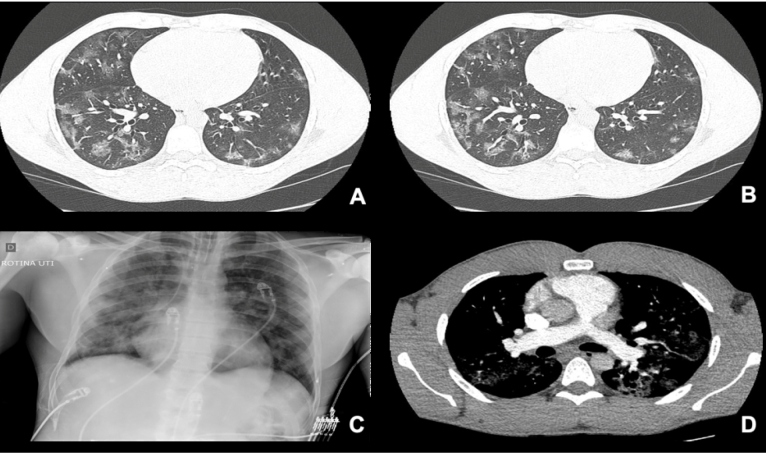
Images obtained at admission. CT scan demonstrating multiple lobe ground-glass opacities (A and B), chest radiograph with peripheral opacities (C), and CTPA with no signs of thromboembolism (D). CT: computed tomography; CTPA: computed tomography pulmonary angiogram.
